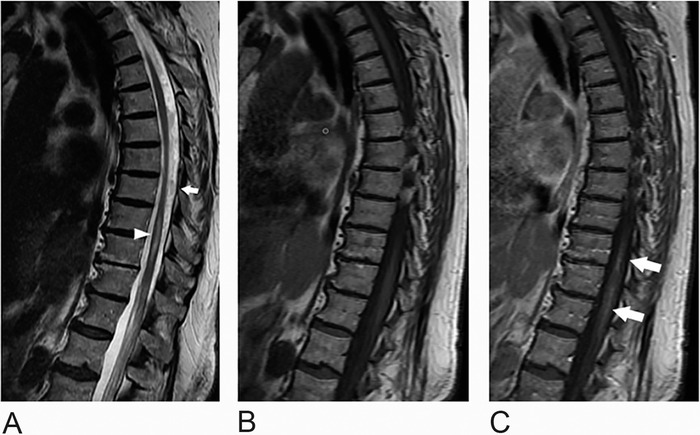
Magnetic resonance imaging of the thoracic spine 14 months postembolization. (A) Sagittal T2-weighted image demonstrates persistent but considerably decreased patchy central cord T2 hyperintensity extending from the T8 level to the conus (arrowhead) with decreased prominence of the venous flow voids in the posterior intradural space (arrow). Sagittal T1-weighted images (B) before and (C) after contrast administration demonstrate mild persistent faint enhancement, considerably improved since the initial examination (arrows).
